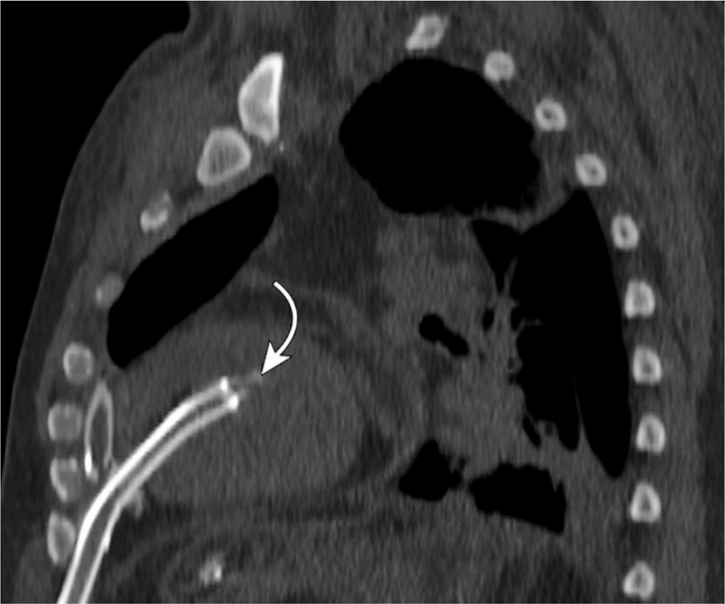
Sagittal reformat from a CT demonstrating a left ventricular drain (arrow) terminating in the left ventricle. This drain aids in decompression when left ventricular function is poor
